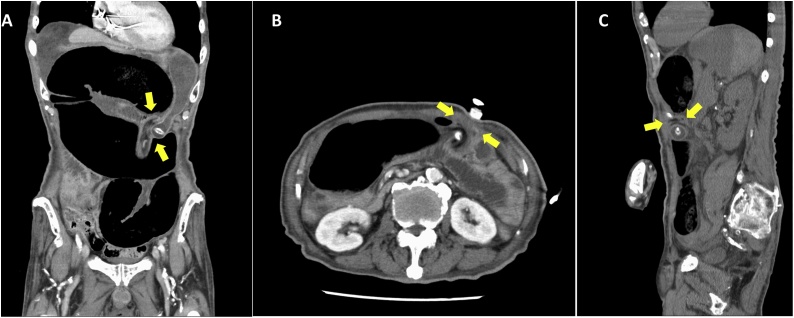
(A) Contrast-enhanced computed tomography revealed internal herniation of the sigmoid colon between the abdominal wall and the stomach as well as the whirling mesentery at the gastrostomy site on the coronal veiw, on the axial view (B), and on the sagittal view (C).
